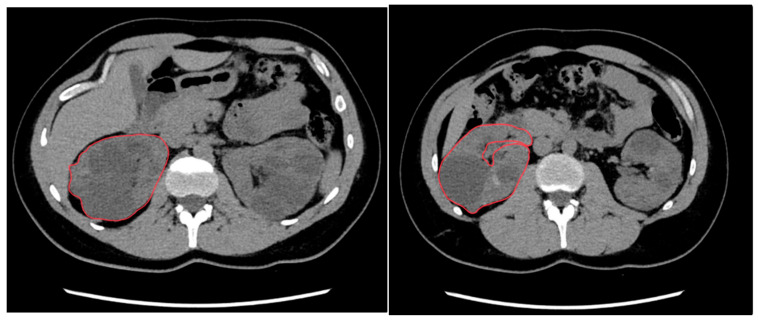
Renal volume calculation using manual segmentation. CT slices from a 45-year-old male patient with autosomal dominant polycystic kidney disease (ADPKD). The operator outlines the kidney contour (in red), excluding the hilar structures.
